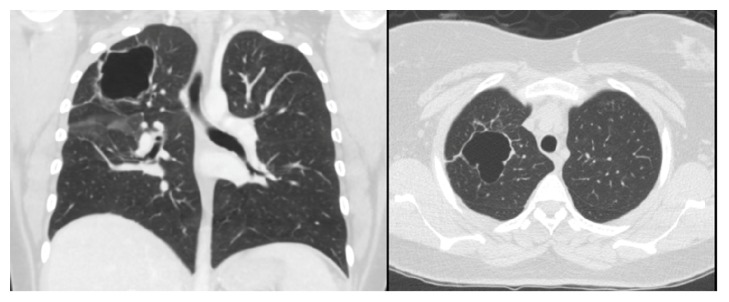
Chest CT on discharge showing resolution of the BPF and development of thin wall right upper lobe cavity.
